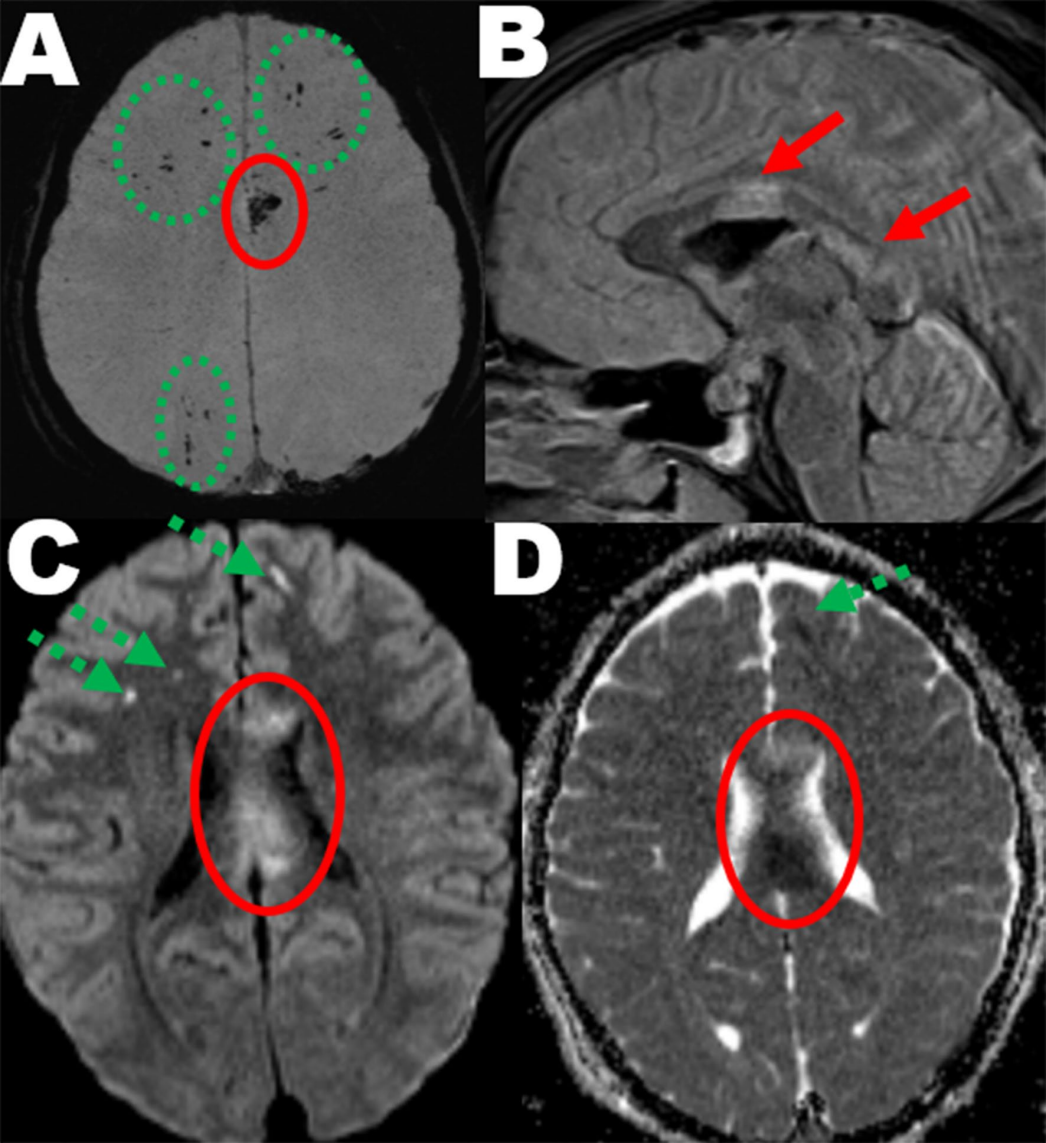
Findings of traumatic axonal injury in three different MRI sequences. A young male was admitted with GCS score 12 to the university hospital after a road traffic accident. CT in the acute phase was normal. 1.5T MRI was performed two days post-injury and showed traumatic axonal injury (TAI): A) Axial susceptibility weighted imaging (SWI) maximum intensity projection showing multiple traumatic microbleeds as sign of micro-haemorrhagic TAI in the hemispheric white matter of both frontal lobes and right parietal lobe (dashed green rings) and corpus callosum (continuous red ring). B) Sagittal FLAIR image showing TAI lesions in truncus and splenium of corpus callosum (continuous red arrows). C) The b1000 image of the diffusion weighted imaging (DWI) also showed hyperintense TAI lesions in the white matter of both frontal lobes (dashed green arrows, also corresponding to the traumatic microbleeds in image A) and corpus callosum (red continuous ring). D) The apparent diffusion coefficient (ADC) image at the same level as the b1000 image (image C) shows low diffusion of both one of the hemispheric TAI lesions (dashed green arrow) and most of the corpus callosum lesion (continuous red arrow)
